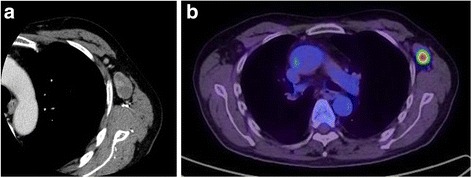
a Axial view of computed tomography showed a 33 × 19 mm enlarged left axillary lymph node (b) ^18^F-fluorodeoxyglucose positron emission tomography revealed abnormal accumulation in the left axillary fossa a maximum standard uptake value of 12.1 and no other metastatic sites
